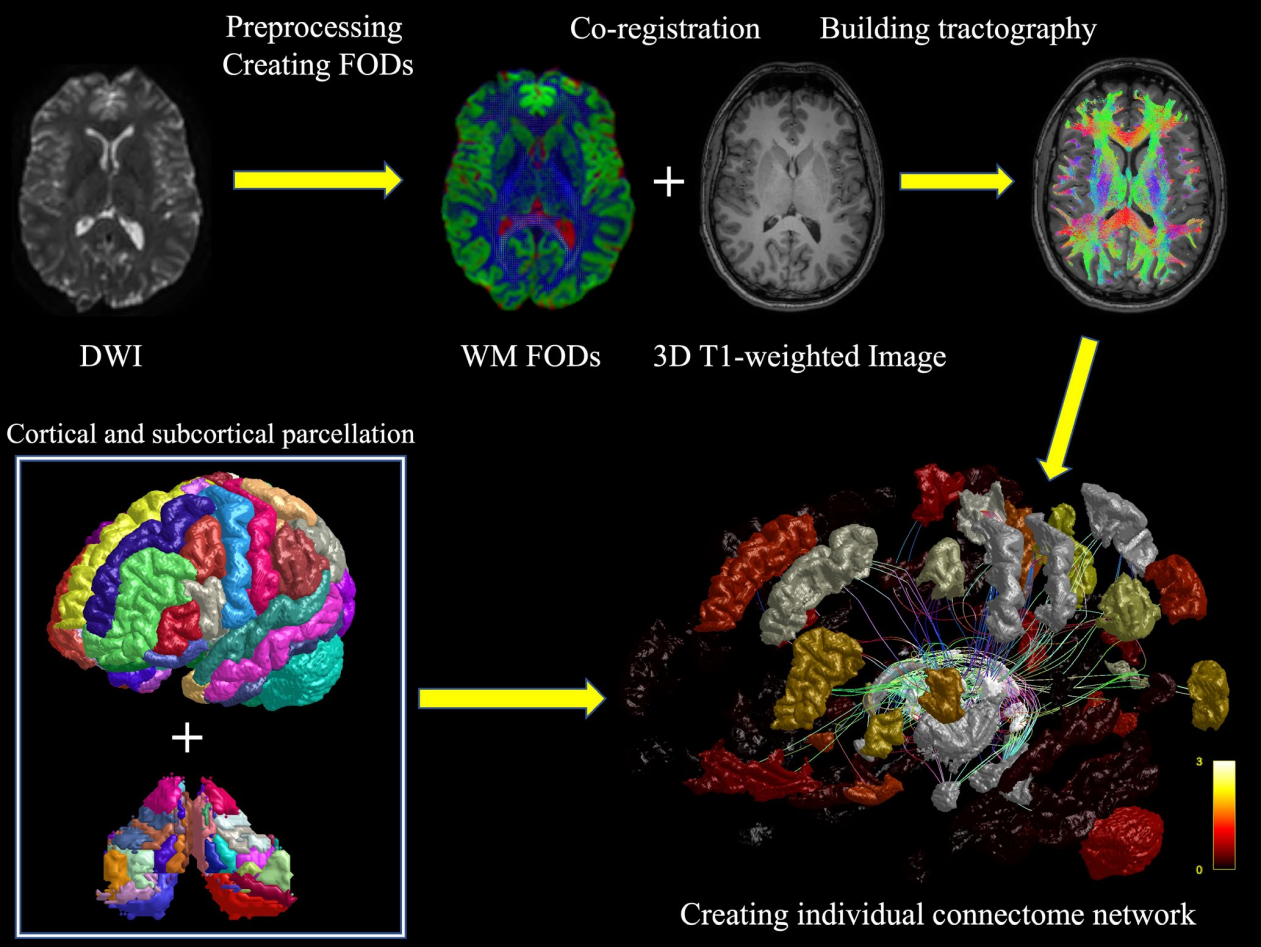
Connectivity analysis was performed using the MRtrix3 software package, FSL, and Freesurfer for cortical and subcortical brain segmentation (see image in the bottom left). First, preprocessing of the diffusion‐weighted imaging (DWI) data was performed, followed by the creation of fiber orientation distributions (FODs). Afterward, co‐registration of the diffusion‐weighted imaging and anatomical T1‐weighted imaging data was performed. The tractography was done by creating 50 million streamlines (see images in the first line). Finally, the connectivity network was built in individual patient's brain based on anatomical segmentation and tractography. The image in the bottom right shows the visualization of the connectivity network of the left‐hemispheric thalamus. Edges (streamlines) are colored by direction (red: Left‐to‐right, commissural fibers; green: Anterior‐to‐posterior, association fibers; blue: Cranial‐to‐caudal, projection fibers). Nodes (brain regions) are colored according to their connectivity strength with the seed point. The colored bar indicates the number of streamlines per volume connecting the seed point with the target region.
